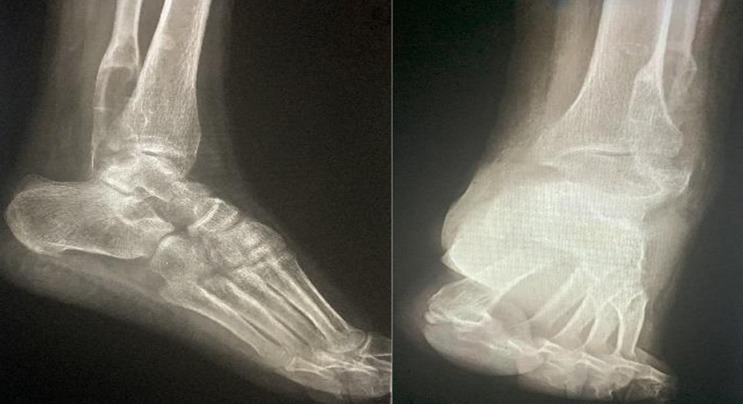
radiographie standard de la cheville gauche montrant une augmentation du volume de la lésion ostéolytique de la malléole externe, sans réaction périostée notable
